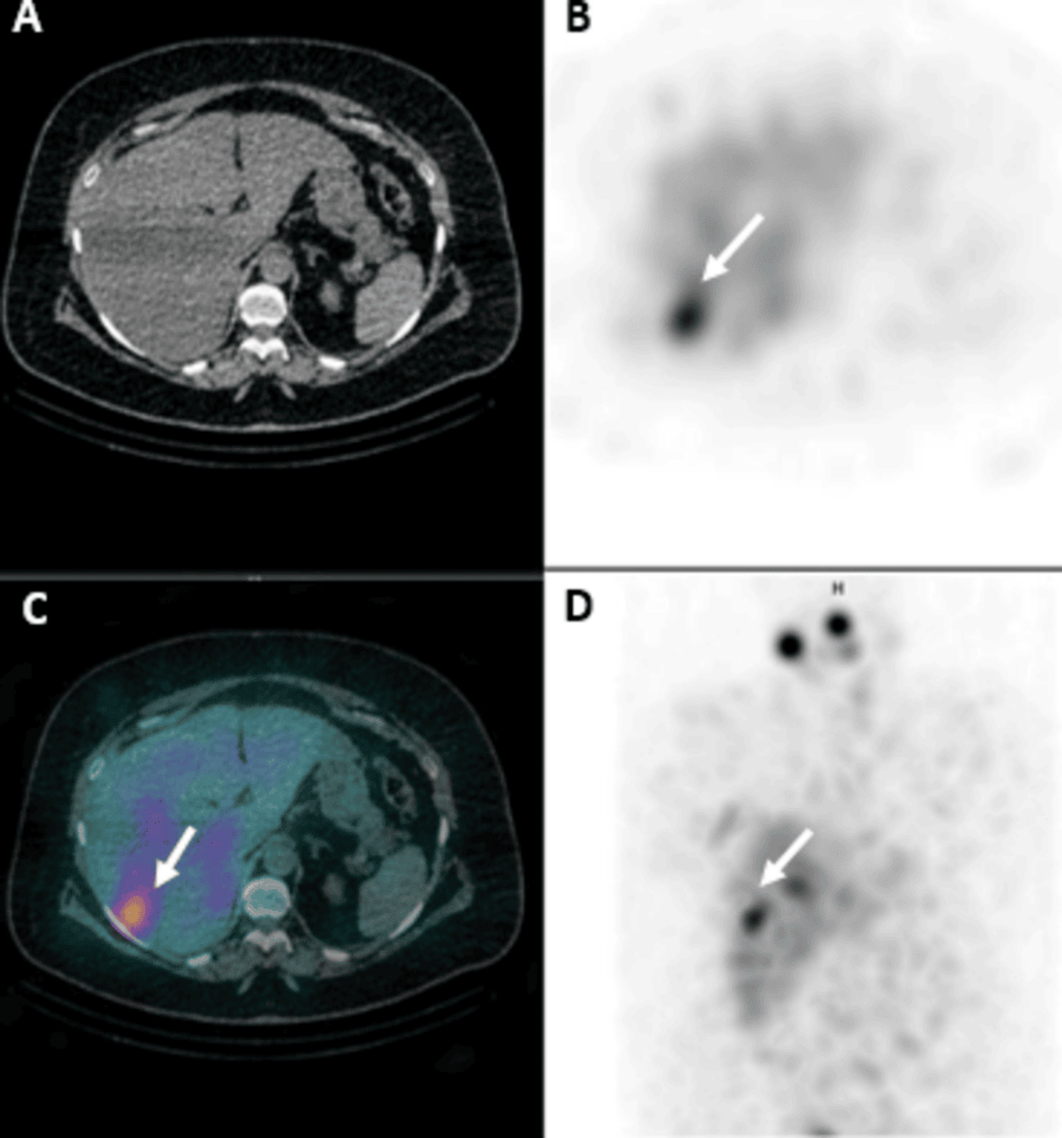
Post-ablation whole-body RAI SPECT/CT (second RAI dose). Post-ablation whole-body RAI SPECT/CT done one week after the second RAI dose shows RAI uptake (white arrow) within hepatic segment VII seen in SPECT, fused, and MIP images (B, C, and D, respectively), not corresponding to definite CT changes (A) with non-specific RAI activity in the remaining liver. RAI, radioactive iodine; SPECT/CT, single photon emission computed tomography/computed tomography; MIP, maximum intensity projection
